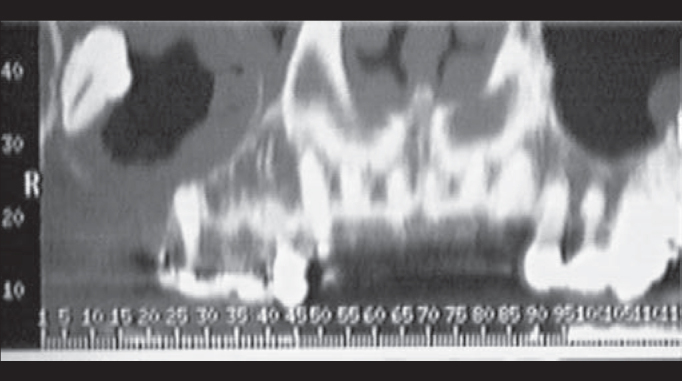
Computed tomography: Right intramaxillary cyst with oroantral fistula.
